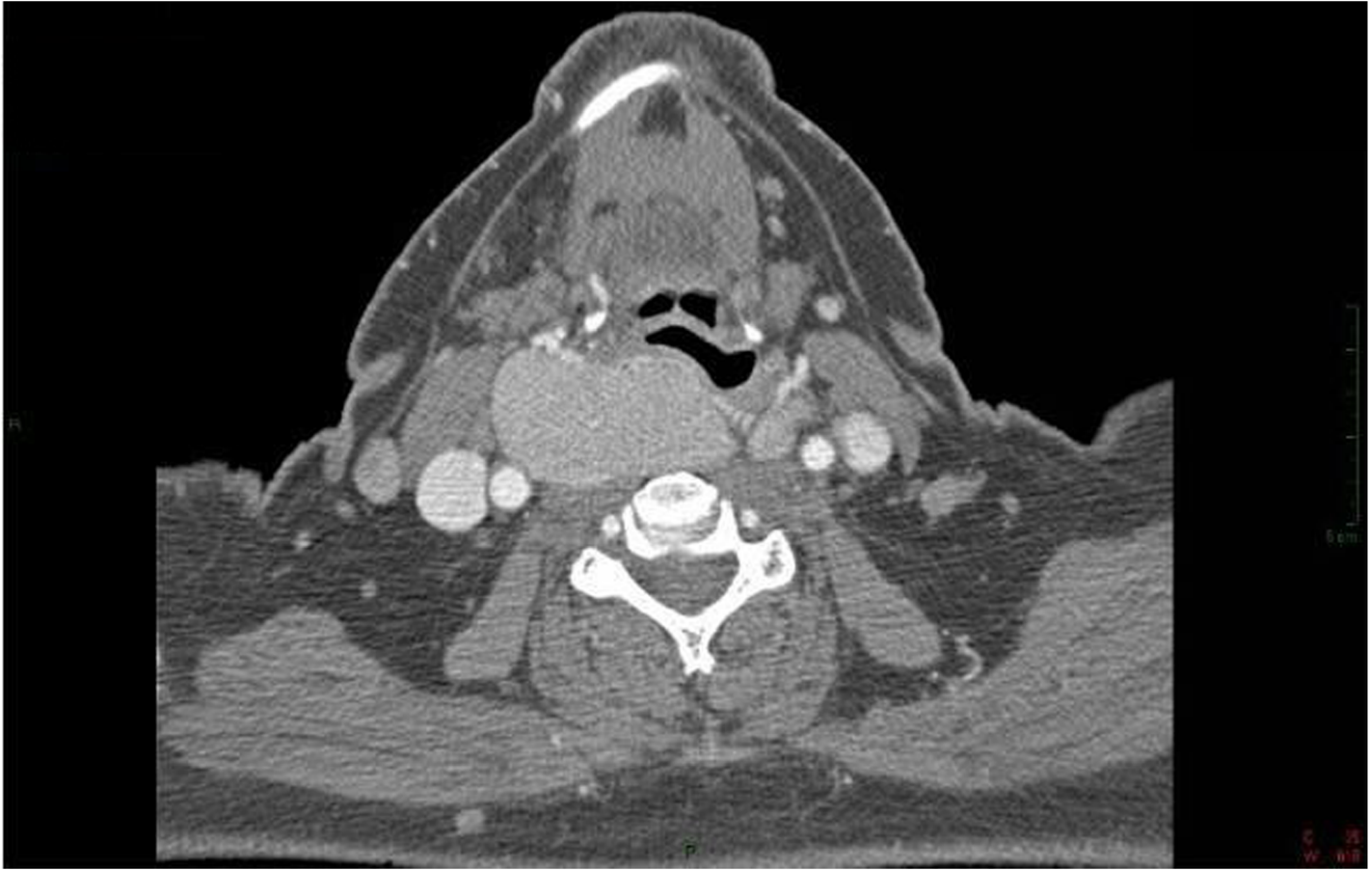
Preoperative computed tomography scan shows the goiter compressing trachea and esophagus.
